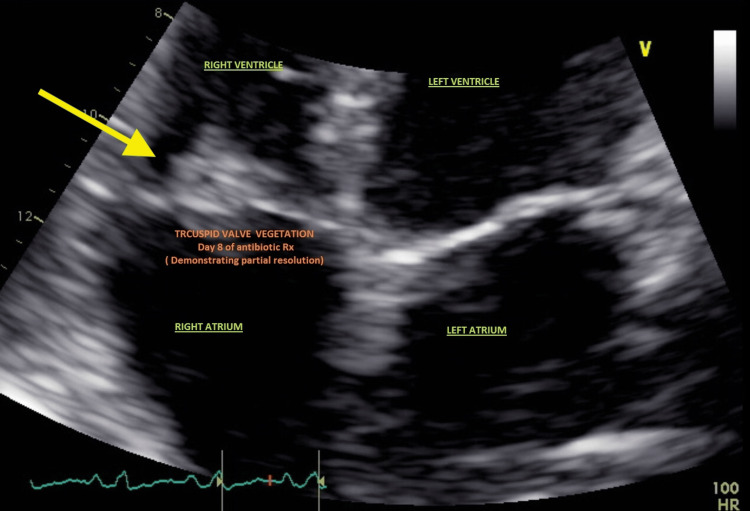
Echocardiogram demonstrating partial resolution of tricuspid valve vegetation after eight days of antibiotic therapy.
